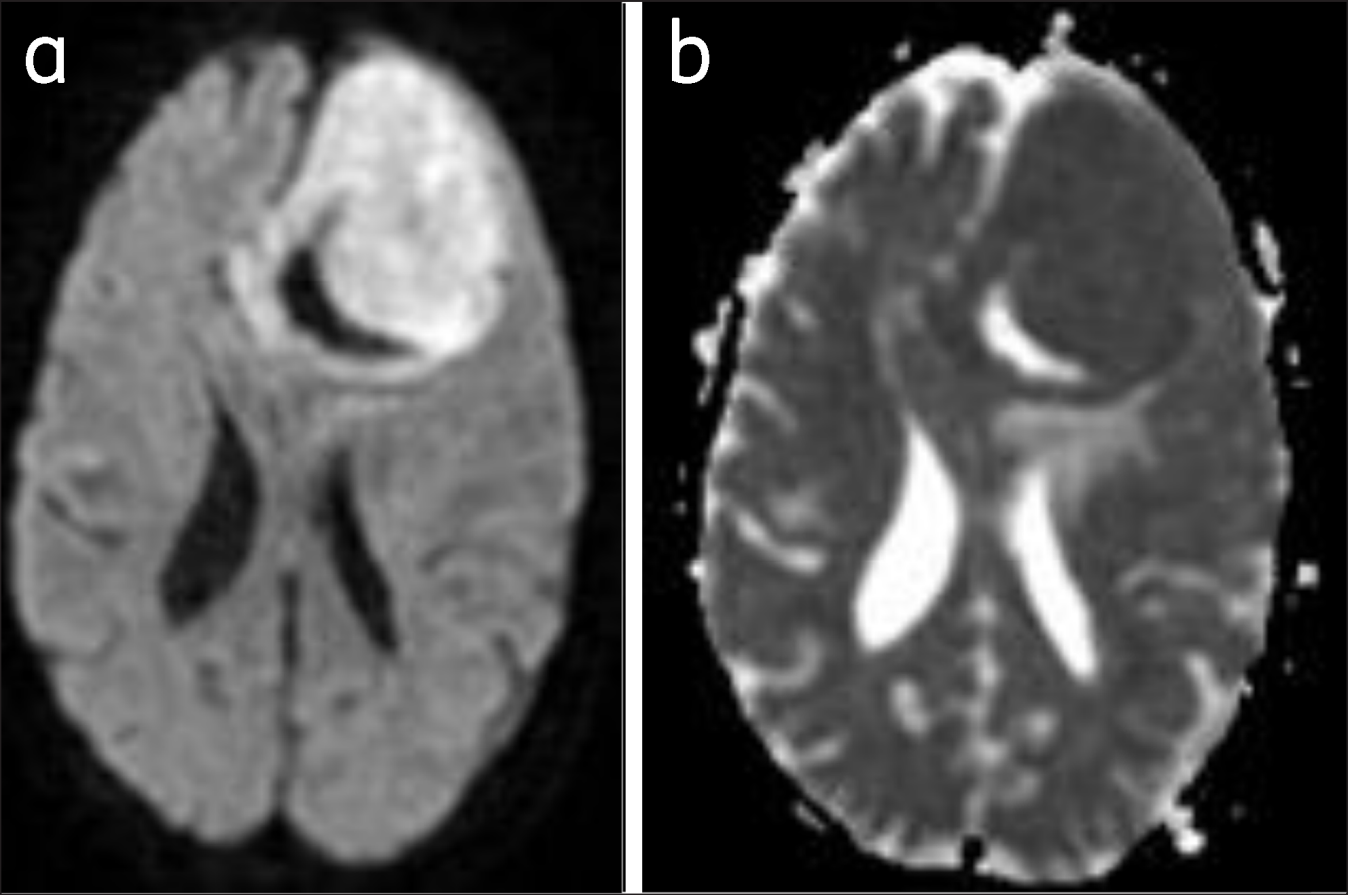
Meningioma with restriction of diffusion: Bright on DWI (a) and dark on ADC (b).
